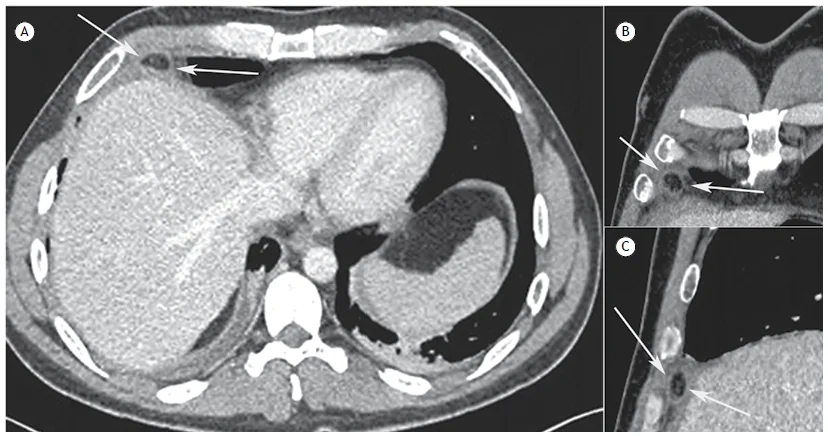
Contrast-enhanced chest CT images (soft-tissue window) obtained in the axial (A), coronal (B) and sagittal (C) planes showing an ovoid fatty lesion in the anterior right costophrenic space, demarcated by a soft-tissue attenuation rim (arrows) and a small right pleural effusion.
